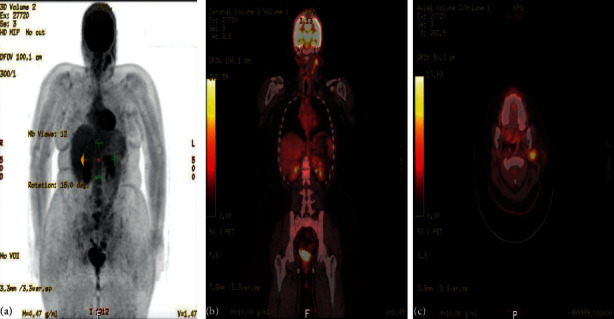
The PET scan in August 2020. The study showed an interval anatomic increase in size and metabolic progression in the known suspicious left deep parotid lesion ((a)–(c)). Measure approximately 2.0 × 1.9 × 2.2 cm in anteroposterior, transverse, and craniocaudal dimensions, respectively, with standard uptake value max (SUVmax) of 3.2. The SUVmax was previously 2.2. On the other hand, there is a more prominent left infraparotid subcentimeter node showing mild fluorodeoxyglucose (FDG) uptake. SUVmax 1.2 is indeterminate. Moreover, an anatomically stable mild FDG-avid left jugulodigastric subcentimeter node with SUVmax up to 1.4 and right supraclavicular node (SUVmax 1.0) are nonspecific, likely reactive. No other size significant FDG-avid cervical lymphadenopathy.
